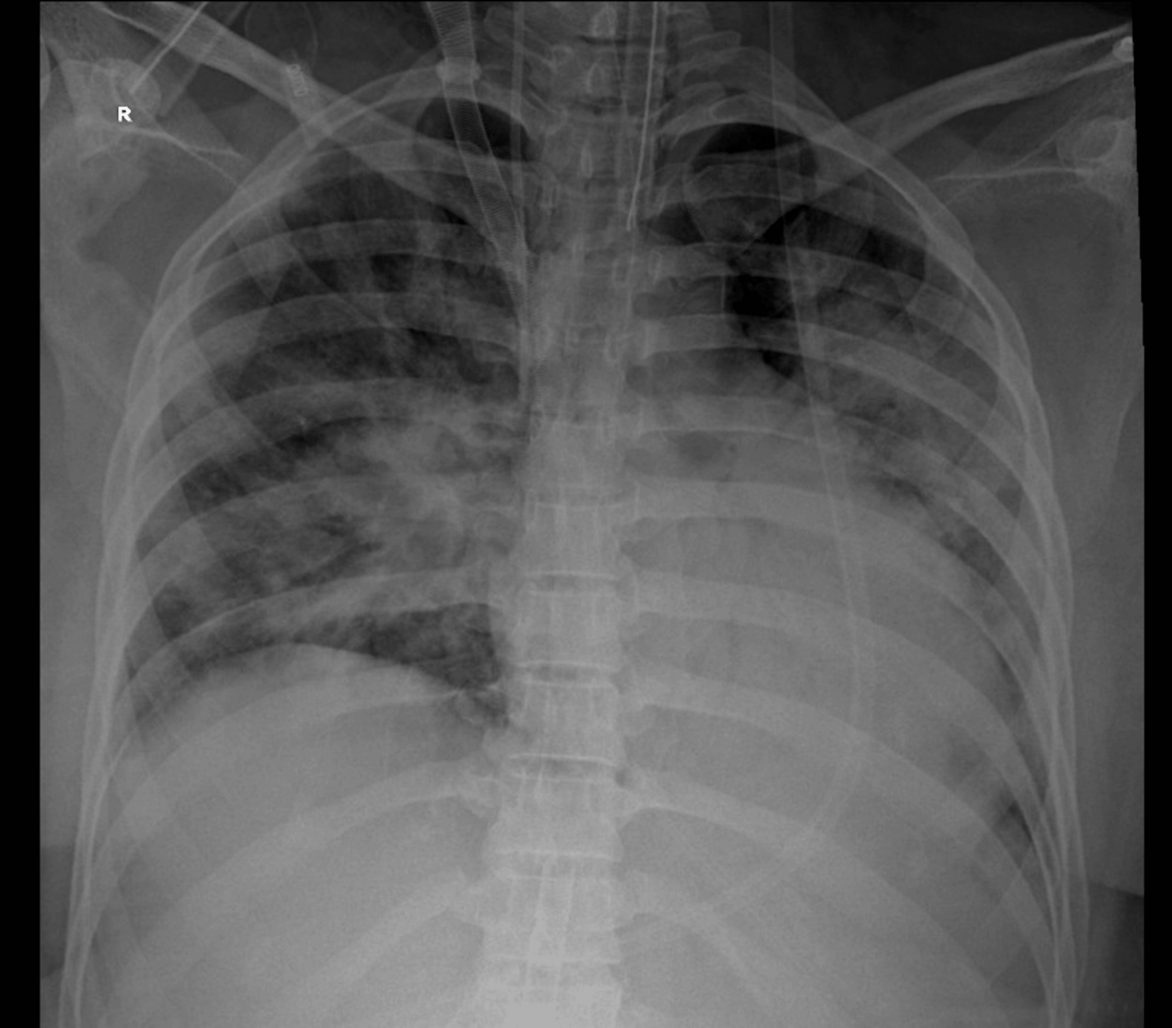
Progressive improvement on chest X-ray after ECMO initiation. ECMO, extracorporeal membrane oxygenation
